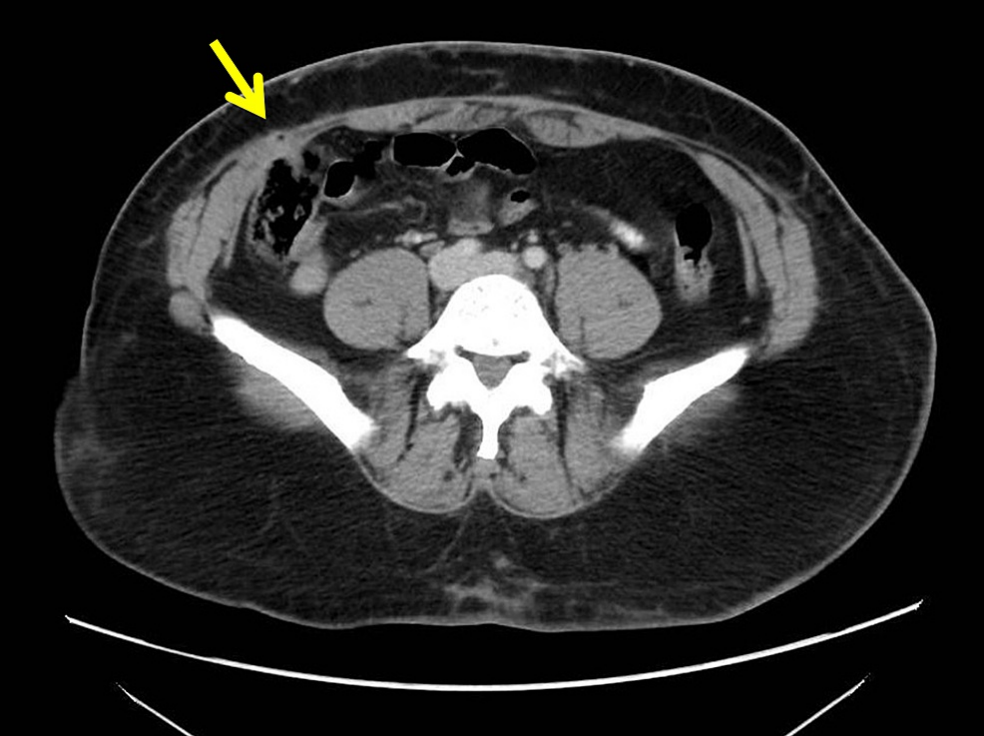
Preoperative CT of abdomen CT image of abdomen preoperatively demonstrating a 1 cm by 1 cm outpouching of hypervascularity through the anterior abdominal wall. This abdominal pathology was not appreciated in the previous CT from the emergency department months prior.
